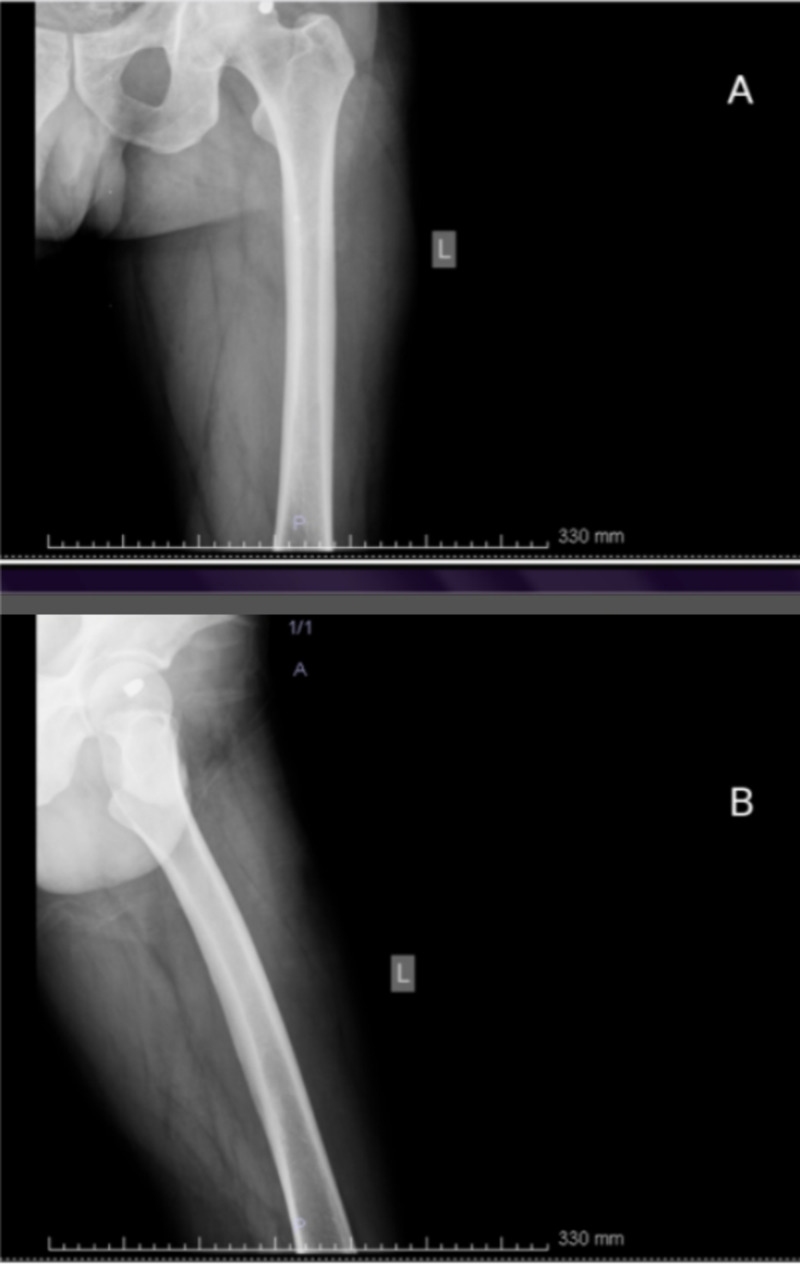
Computed tomographic scans A. Preoperative anteroposterior hip view, B. Preoperative lateral hip view
